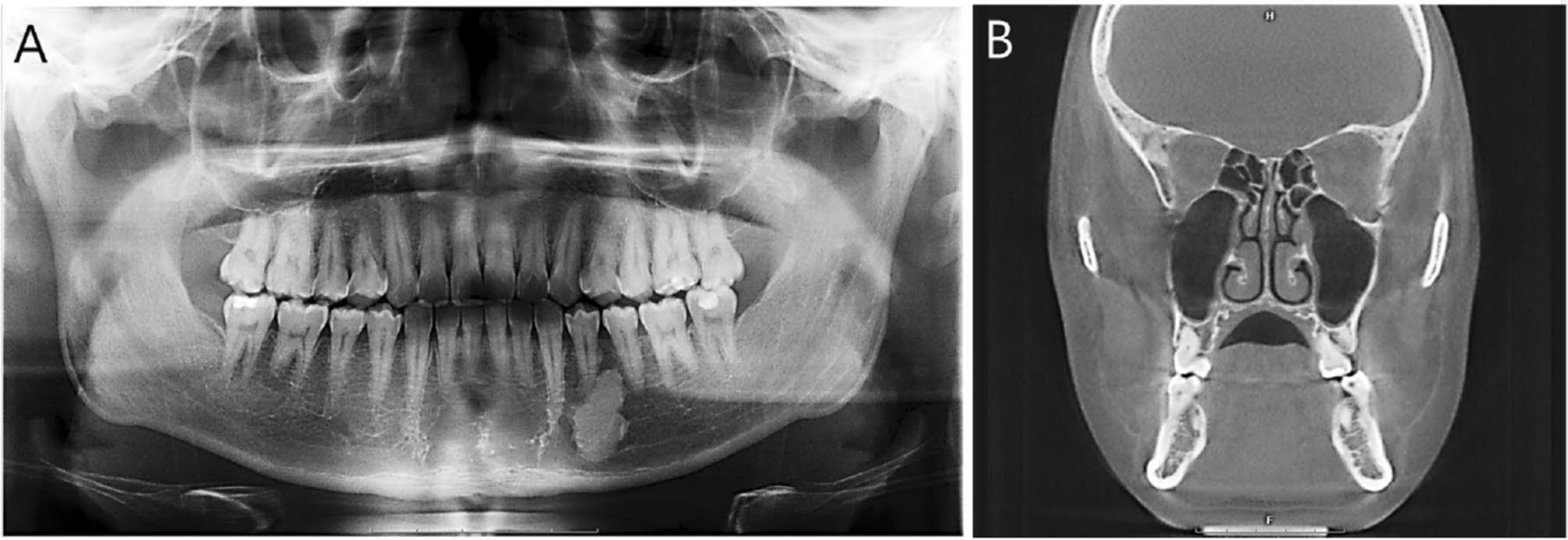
A panoramic image of a 23-year-old male patient seemed to show inflammation of paranasal sinuses based on the haziness of both sinuses, and total opacification was suspected on the right maxillary sinus. However, 3D CBCT image showed clear sinuses without any inflammation. (A) Panoramic radiograph, (B) clear sinuses in a CBCT slice.
